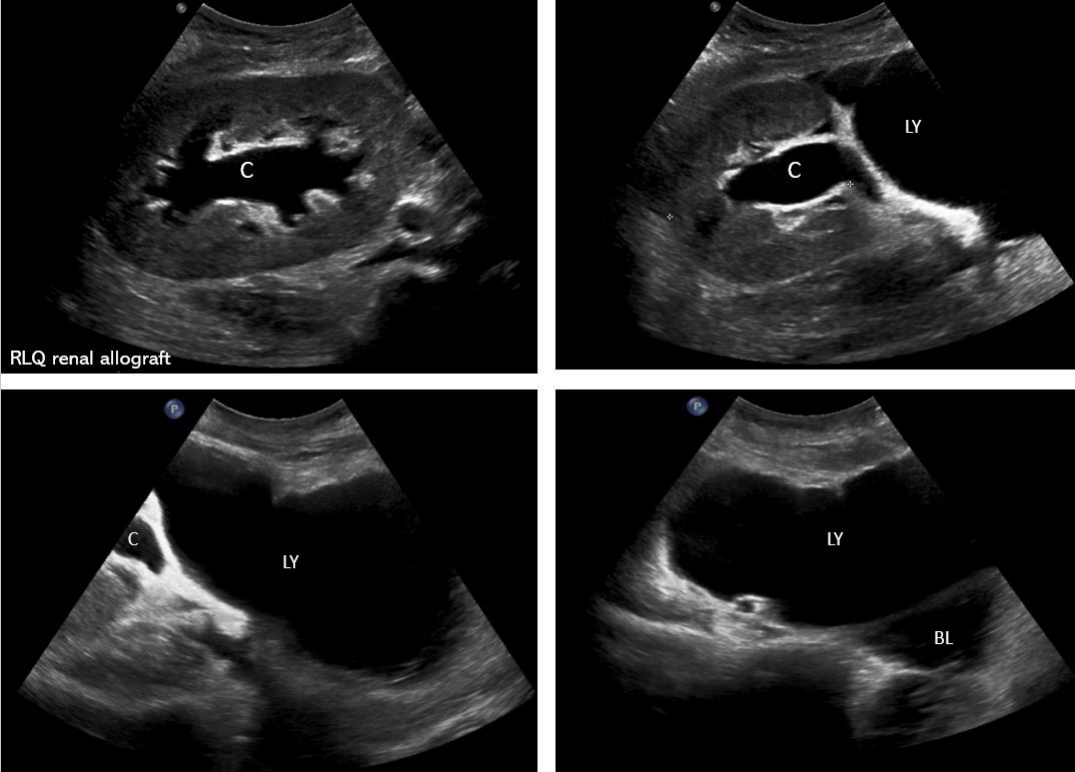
Sonographic images demonstrating a large hypoechoic collection (lymphocele) compressing the ureter and leading to hydronephrosis. Note the relationship between the lymphocele, renal collecting system, and the urinary bladder. RLQ = right lower quadrant; LY = lymphocele; C = renal collecting system; HY = hydronephrosis; BL = urinary bladder.
